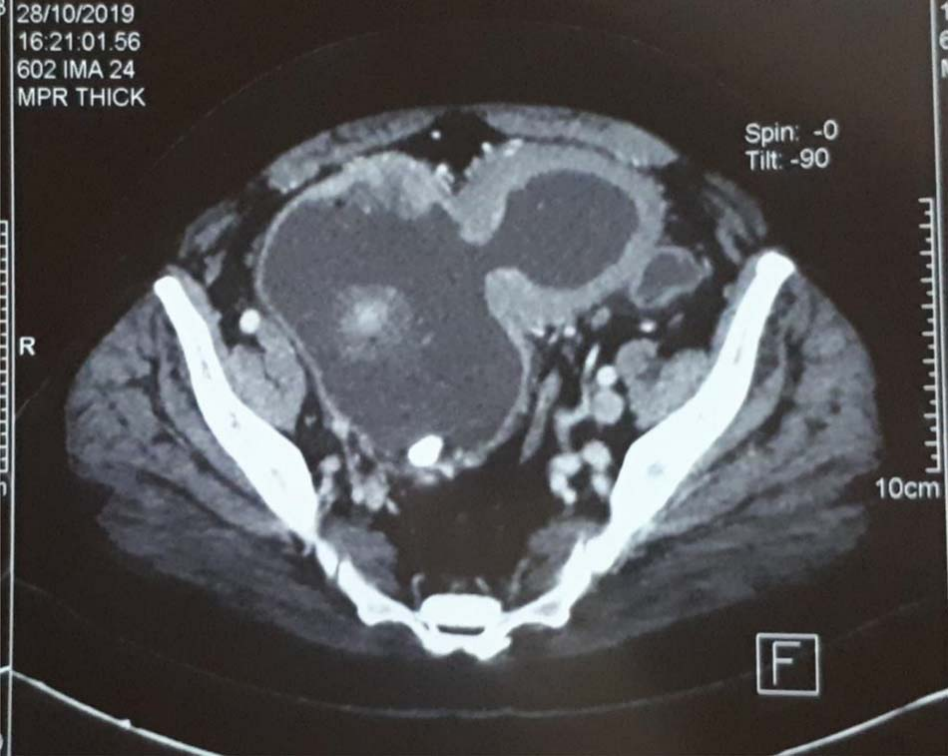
CT-scan of the bladder 2 months before the rupture showing bladder tumor in the dome and in intradiverticular and a bladder stone.
